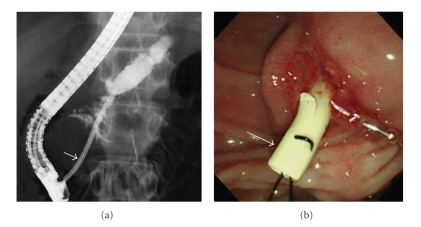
(a) X-ray image, (b) endoscopic image. The stent was transpapillarily replaced with a 10 F straight stent (arrow) one week later.
